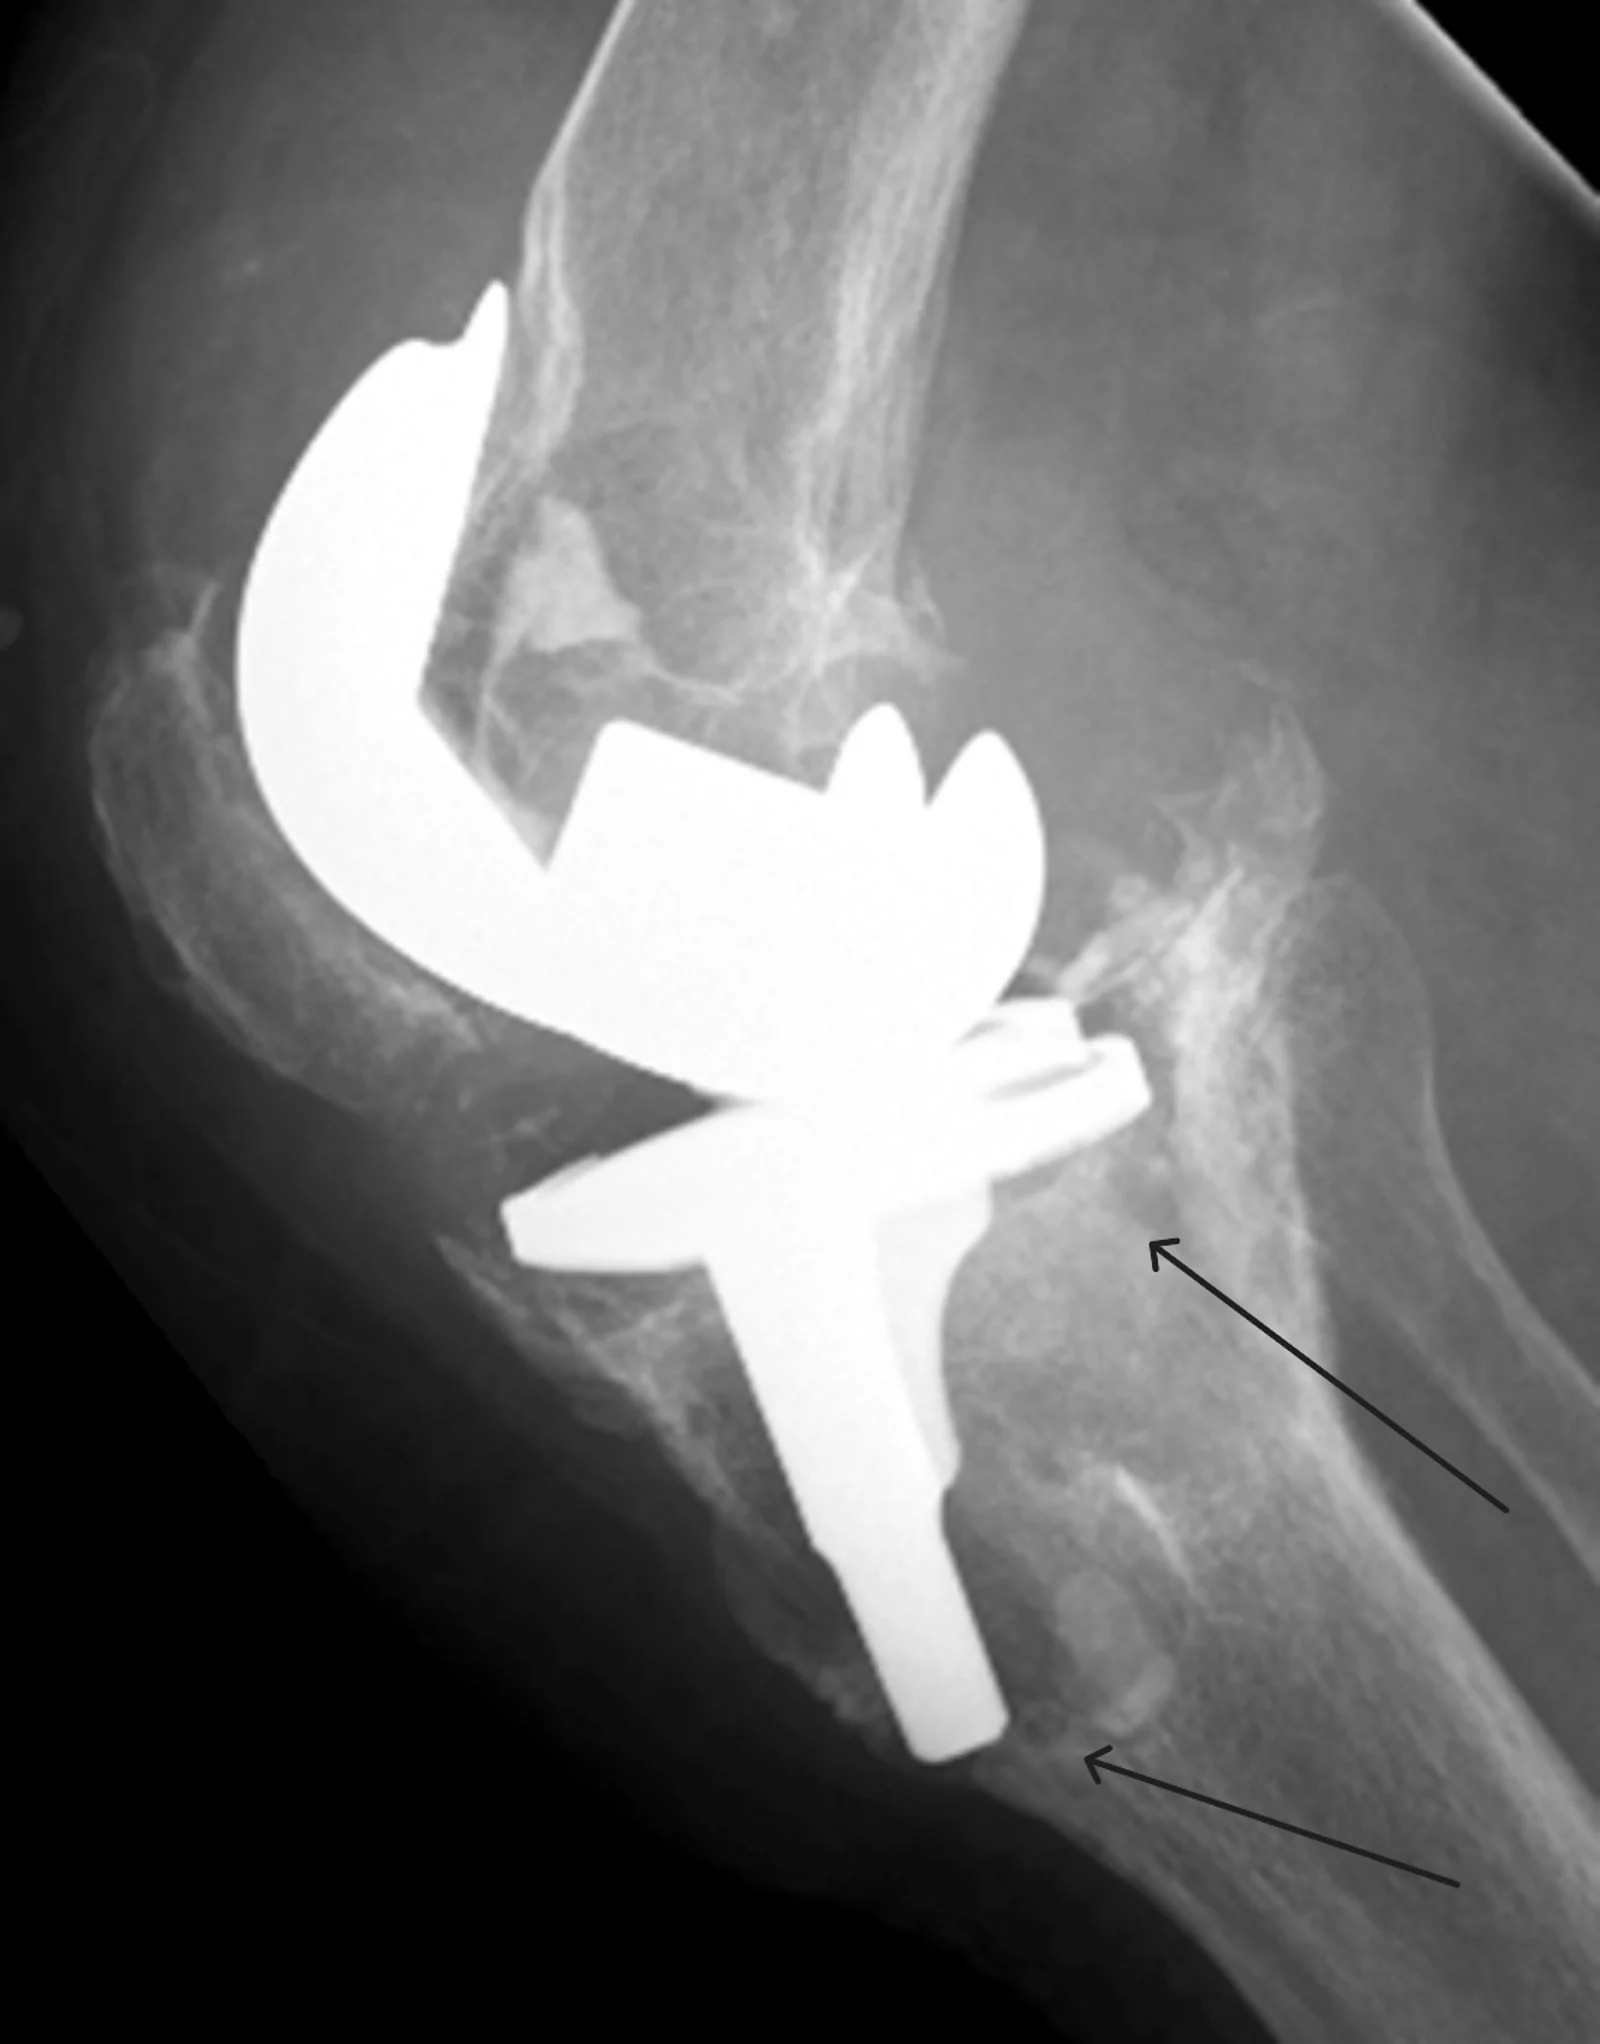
Lateral radiograph of the right knee showing osteolysis of the proximal tibia approximately nine years after primary TKA. TKA, total knee arthroplasty
